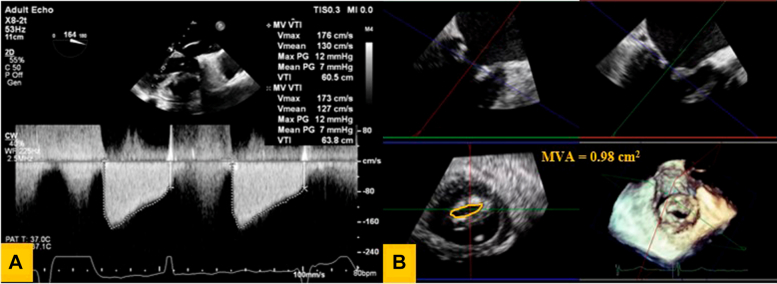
Transesophageal Echocardiography Demonstrating Severe Mitral Stenosis, Patient 2 (A) Mean transmitral gradient of 7 mm Hg. (B) Mitral valve area (MVA) of 0.98 cm^2^ measured by 3-dimensional planimetry.
